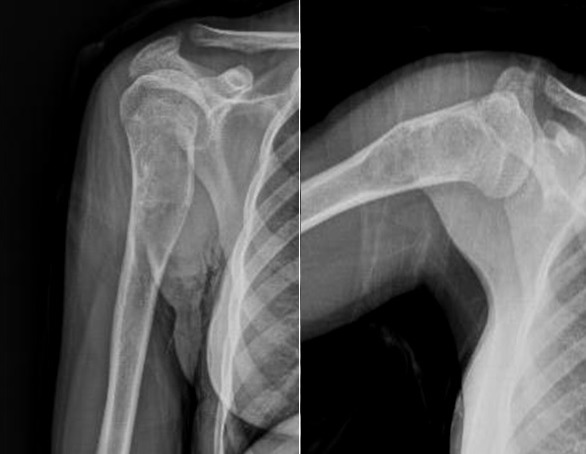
Radiography view of the simple bone cyst of the proximal humeral in 13 year old boy
